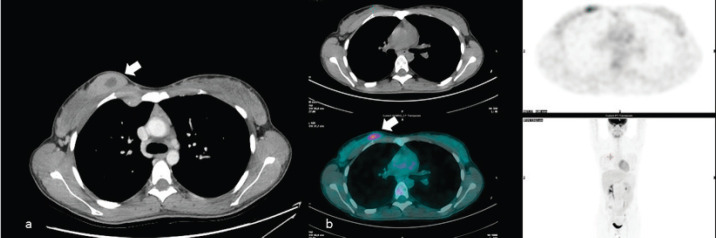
A 50-year-old patient presenting a right unilateral, incidental finding of breast mass subsequently demonstrated to be a MS. (a): Irregular breast mass on the computed tomography exam, showing inhomogeneous contrast enhancement (arrow). (b):The abnormal glucose uptake in PET examination (arrow).
